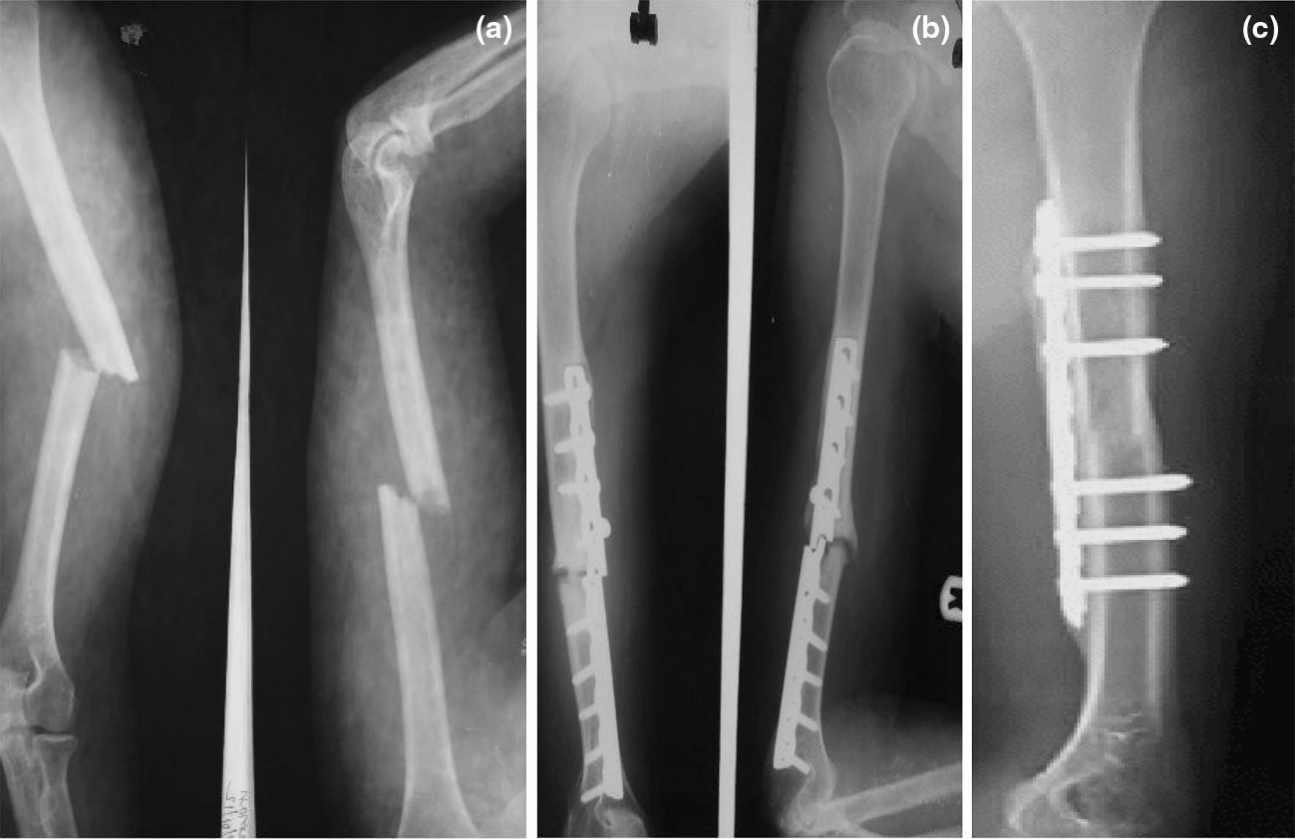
a Preoperative X-ray of a 45-year-old male patient showing a displaced fracture of the shaft of the humerus, right side. b Postoperative X-ray at 6-month follow-up showing implant (LCDCP) failure and nonunion at fracture sites. c Revision surgery (implant removal, freshening of fracture edges, internal fixation with 4.5-mm LCP) resulted in uneventful union of fracture site
